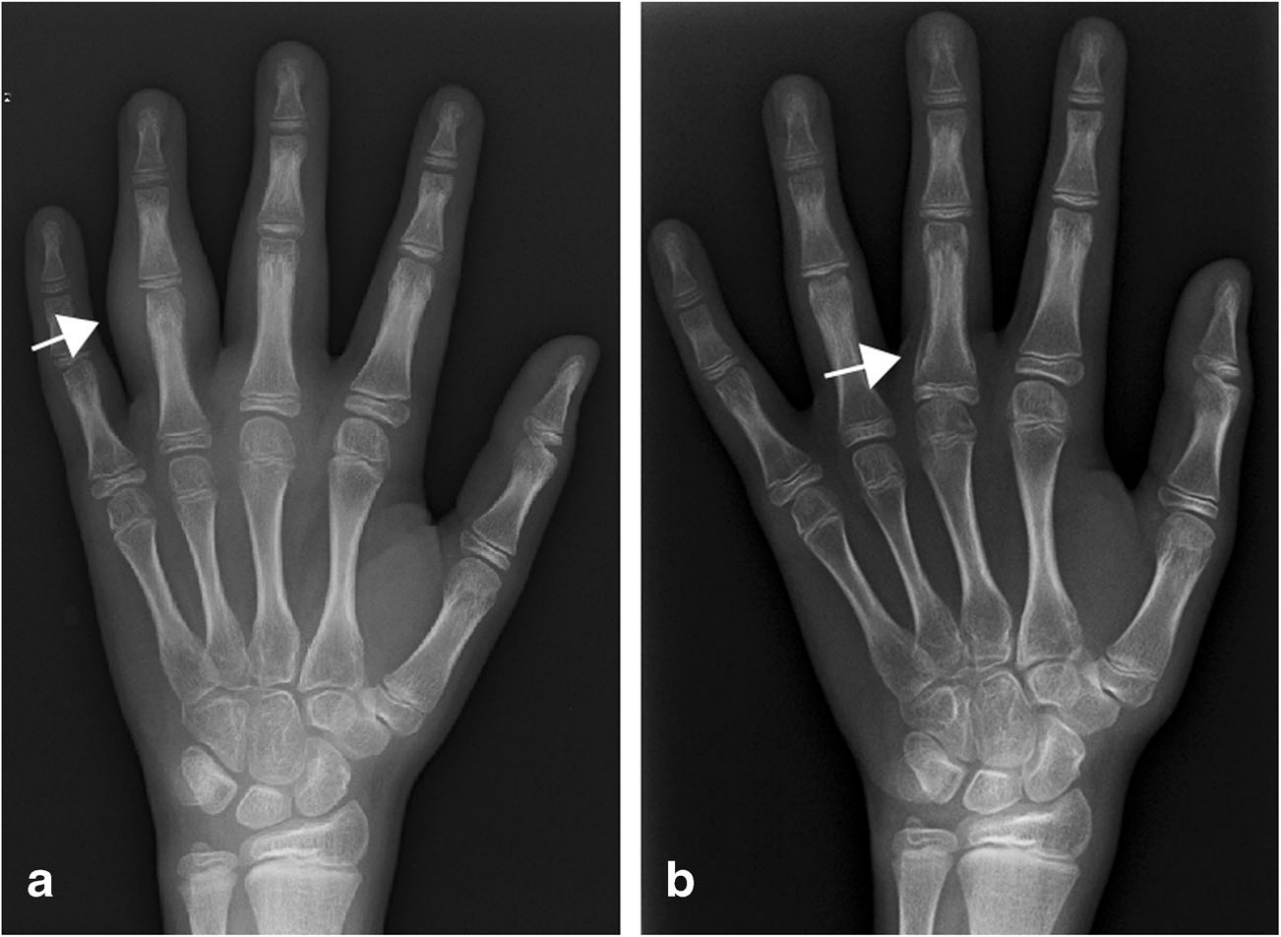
Plain radiographs of affected joints in JIA may be normal, particularly early in the disease course; however, non-specific soft tissue swelling may be seen (a). Periosteal reaction (b) may also occur in active synovitis and is most frequently seen in tenosynovitis.
